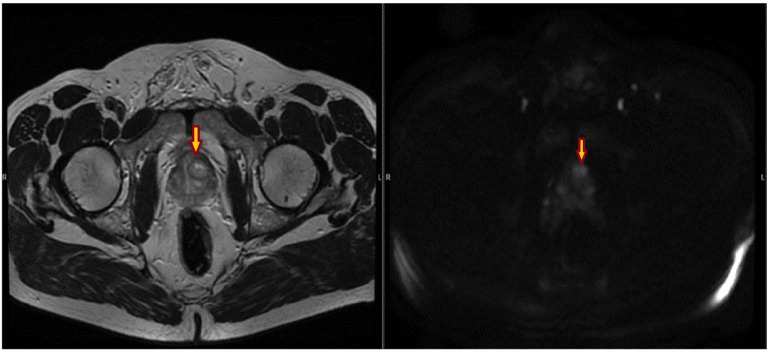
T2 and DWI sequences, showing an uncircumscribed lesion, with restricted diffusion, localized in the anterior transitional zone (left)—yellow arrow. The lesion was scored PI-RADS 5 by all of the radiologists.
